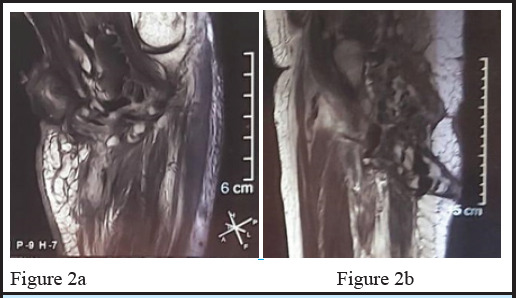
MRI of left elbow showed multiloculated collection with fluid. Figure 2b: MRI of right thigh showed showed sinus tract opening in lateral and posterior aspect of thigh, multiloculated collection with fluid.
